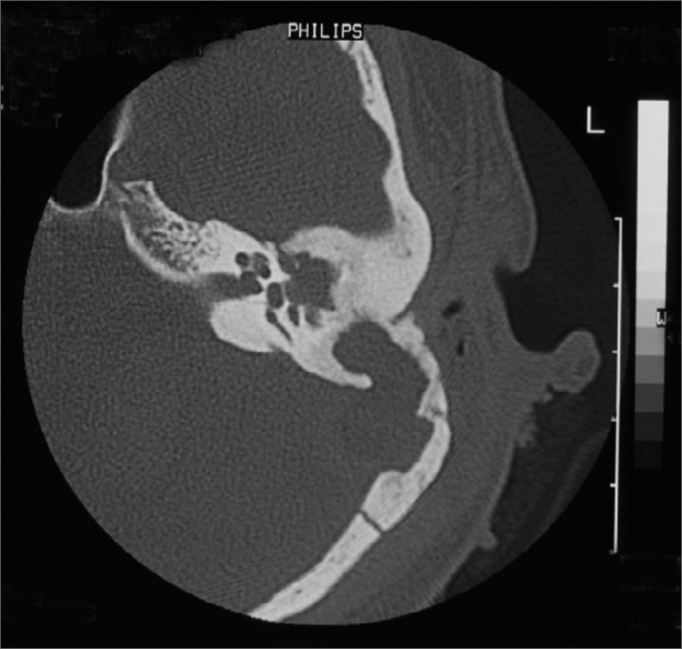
Temporal bones CT scan without contrast, showing extensive bone destruction in the sigmoid sinus area (dura mater of the posterior fossa).
